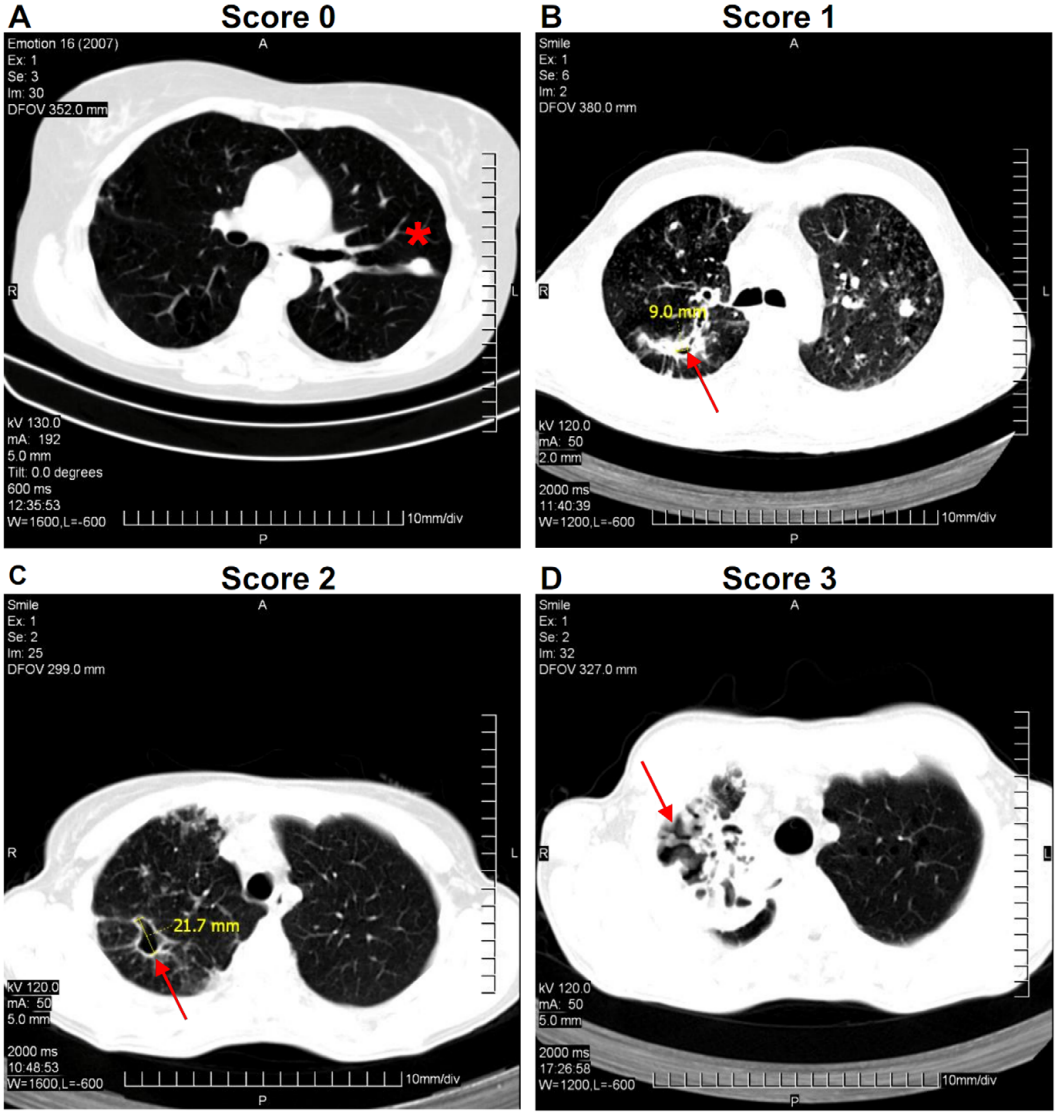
Evaluation of lung tissue destruction. Examples of X-ray computer tomograpy with different degrees of tissue destruction are shown. Lung destruction was evaluated based on the number and size of destructive (lucent) foci (see Methods for the details). A, score 0, no destruction; B, score 1, one small (<2 cm) destruction; C, score 2, one large (>2 cm) destruction; D, score 3, multiple lung destructions. Arrows, lung destructions. Asterisk, infiltrative focus without destruction.
